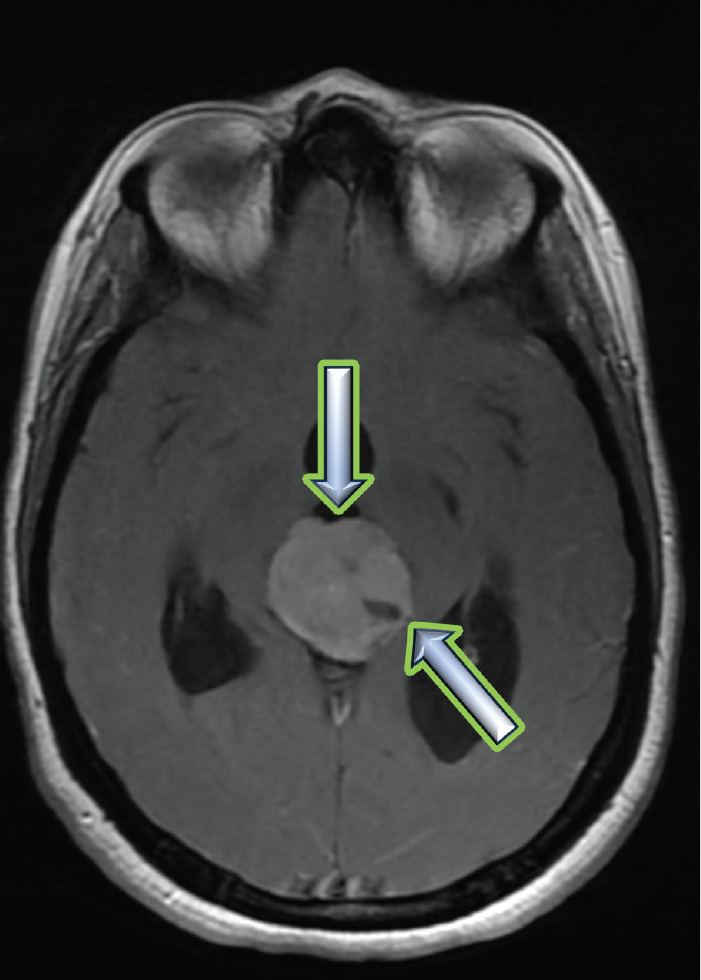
Brain MRI 3 months after RT treatment, showing an estimated response of 38% on a T2 flair sequence.
